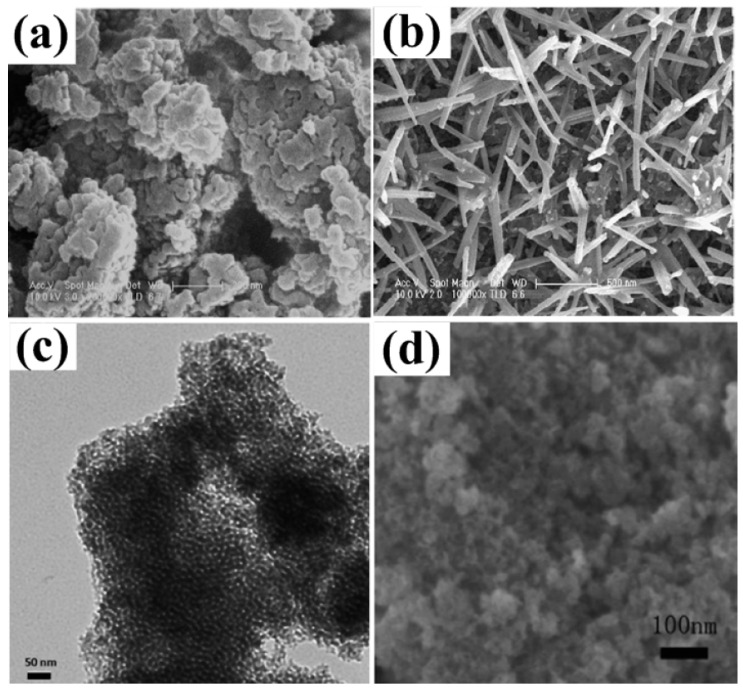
SEM images of MnO_2_ samples prepared using a sonochemical technique at two different amplitudes: (a) 30 m and (b) 60 m. Adapted with permission from Ref. [176]. ©2012, Material Science & Engineering TEM (c) and SEM (d) images of mesoporous MnO_2_ generated by the reaction of potassium permanganate and ethanol under ultrasound irradiation. Reproduced with permission from Ref. [170]. ©2014, Mat letter.
